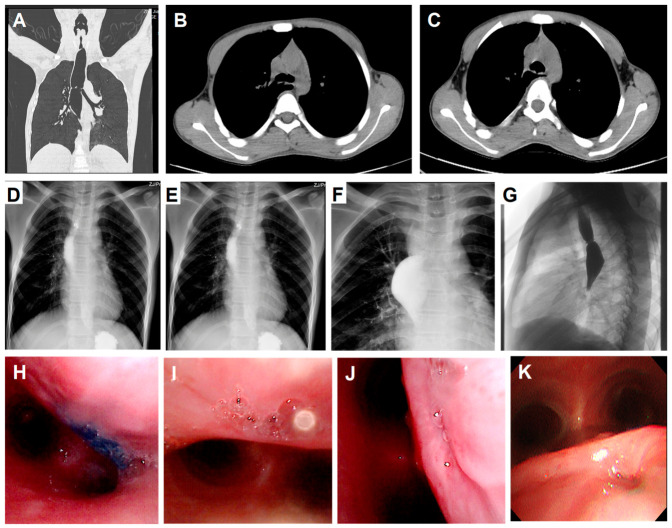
Serial radiological and bronchoscopic findings of the recurrent tracheoesophageal fistula (rTEF) across the treatment course. (A) Initial three-dimensional coronal CT reconstruction in January 2024 demonstrating the fistulous tract between the anterior esophageal wall and the posterior trachea. (B) Axial CT in March 2024 confirming a persistent fistulous tract after the failed endoscopic clipping. (C) Axial CT in September 2025 showing the persistent rTEF after the second open thoracotomy. (D,E) Serial esophagograms after esophageal-side clip closure showing retained titanium clips adjacent to the esophageal opening, localized esophageal narrowing, and persistent contrast leakage through the fistula into the right bronchial tree. (F) Esophagogram after the second thoracotomy demonstrating massive contrast extravasation into the airways, indicating surgical failure. (G) Lateral esophagogram obtained 2 weeks after bronchoscopy-guided intratracheal suture repair, showing no contrast leakage. (H) Initial bronchoscopy showing methylene blue dye leakage from the fistula orifice on the posterior membranous wall after instillation through a nasogastric tube. (I) Bronchoscopy after esophageal-side clip closure showing persistent bubbling secretions from the tracheal opening above the carina, indicating continued fistula patency. (J,K) Serial bronchoscopies before and after the second thoracotomy still demonstrating the persistent fistula with continuous leakage of white foamy liquid.
